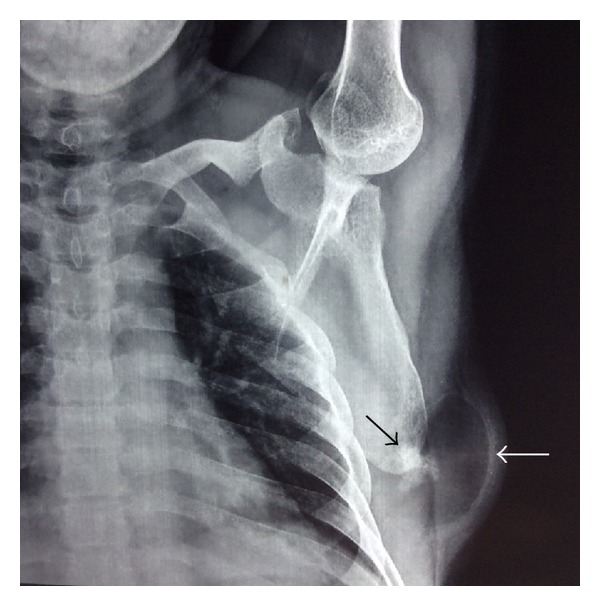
Plain radiograph of left shoulder joint showing a radiolucent lesion (white arrow) with underlying irregular osseous protuberance (black arrow) arising from the inferior tip of scapula.
